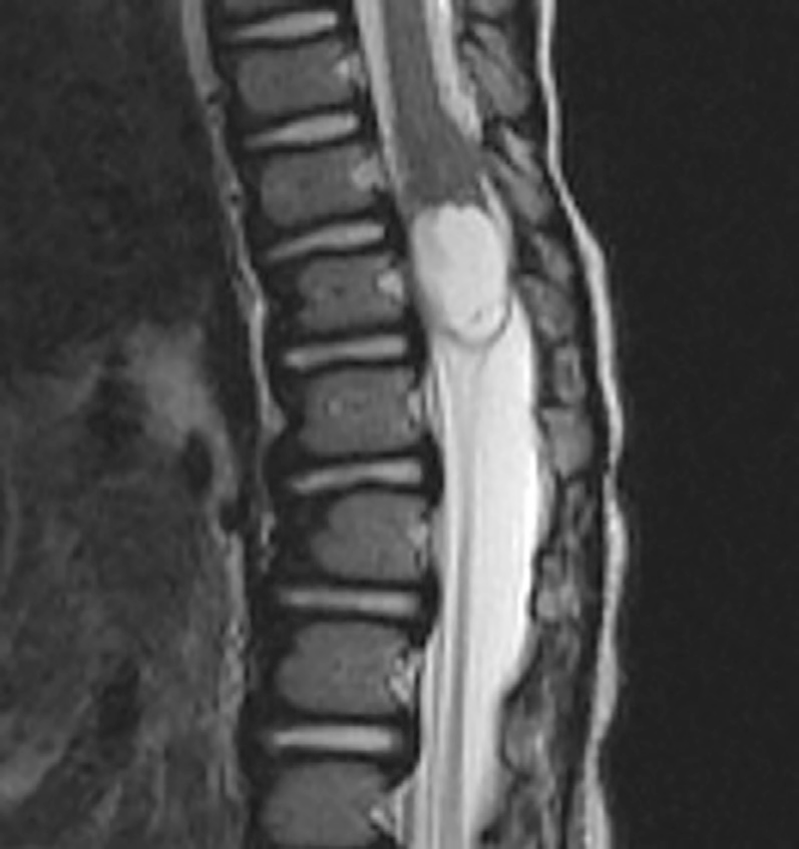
Magnetic resonance imaging analysis Shown is sagittal T2-weighted magnetic resonance imaging of the thoracolumbar spine, demonstrating an expansile, mixed solid and cystic mass centered in the conus at T12.
